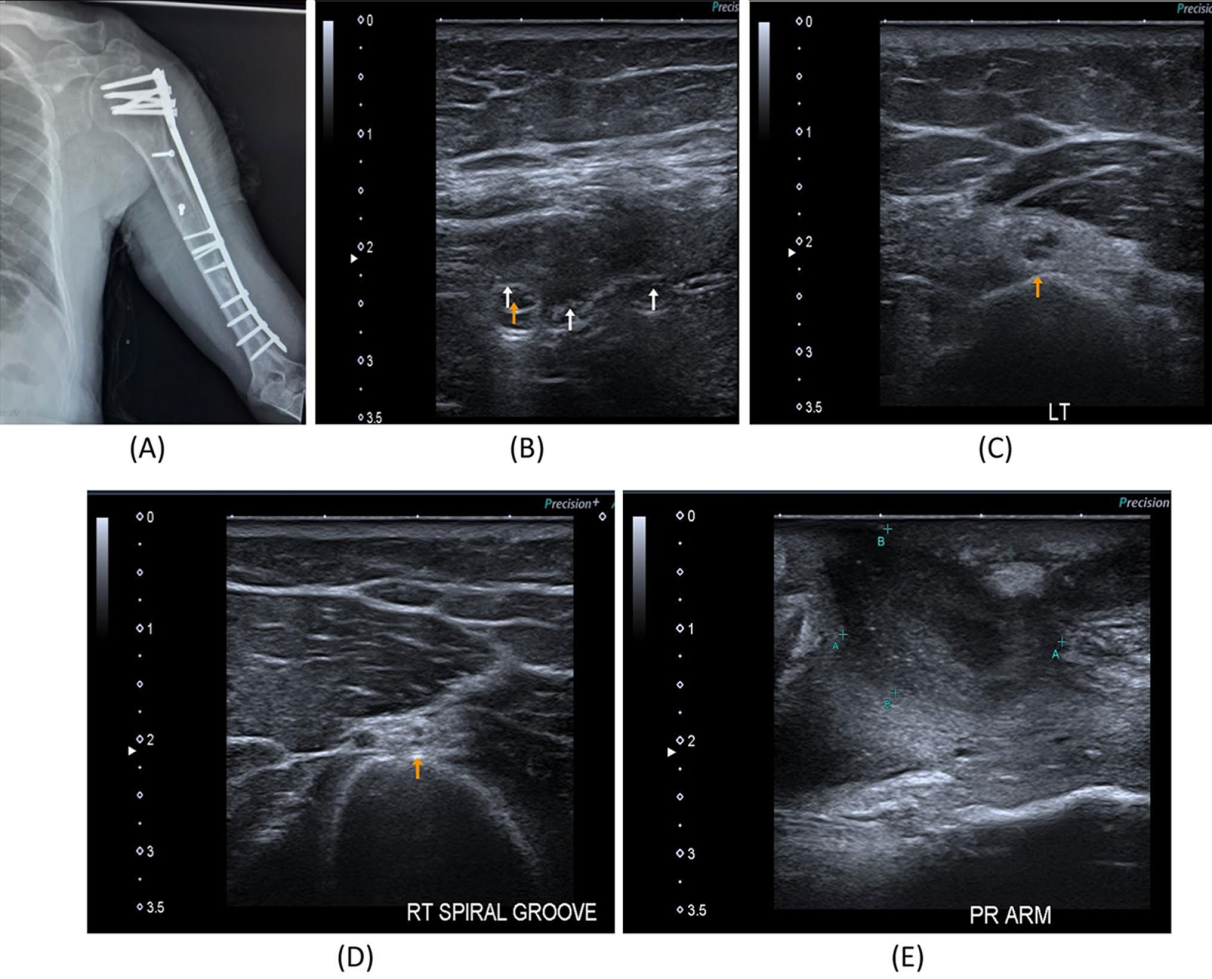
A 35-year- old male with a history of left humeral fracture with internal fixation with plate and screws since July 2021 with a weak extension of the elbow. A Digital radiography image of the left arm showing internal fixation with plate and screws. B B-mode image, hypo-echogenicity saw along the course of the radial nerve within the spiral groove intimately related to applied screws. C B-mode image, transverse axis, thickened left radial nerve in the spiral groove of the humerus, suggested axonal injury proved by NCS. D B-mode image, transverse axis of the right normal radial nerve within the spiral groove of the humerus. E B-mode image, turbid collection noted the lateral aspect of the axilla
